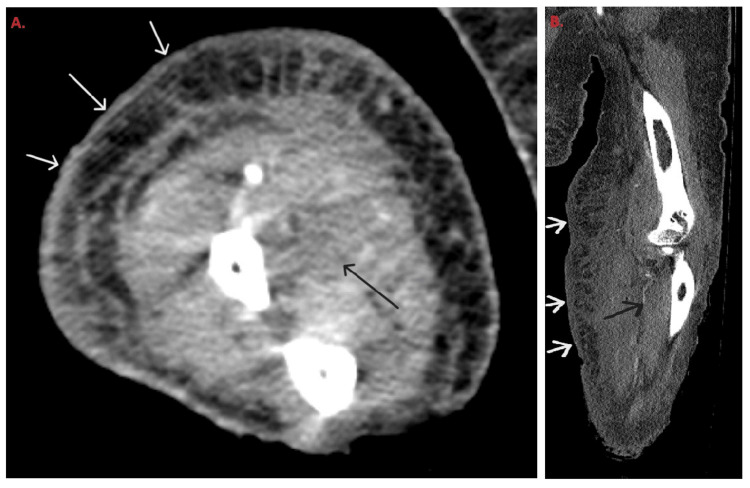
Computed tomography angiography of the upper extremity axial slice through the mid-forearm (A) and sagittal multiplanar reformat (B) demonstrate ulnar arterial occlusion (black arrows) and diffuse cutaneous irregularity (white arrows) consistent with clinically evident skin lesions
